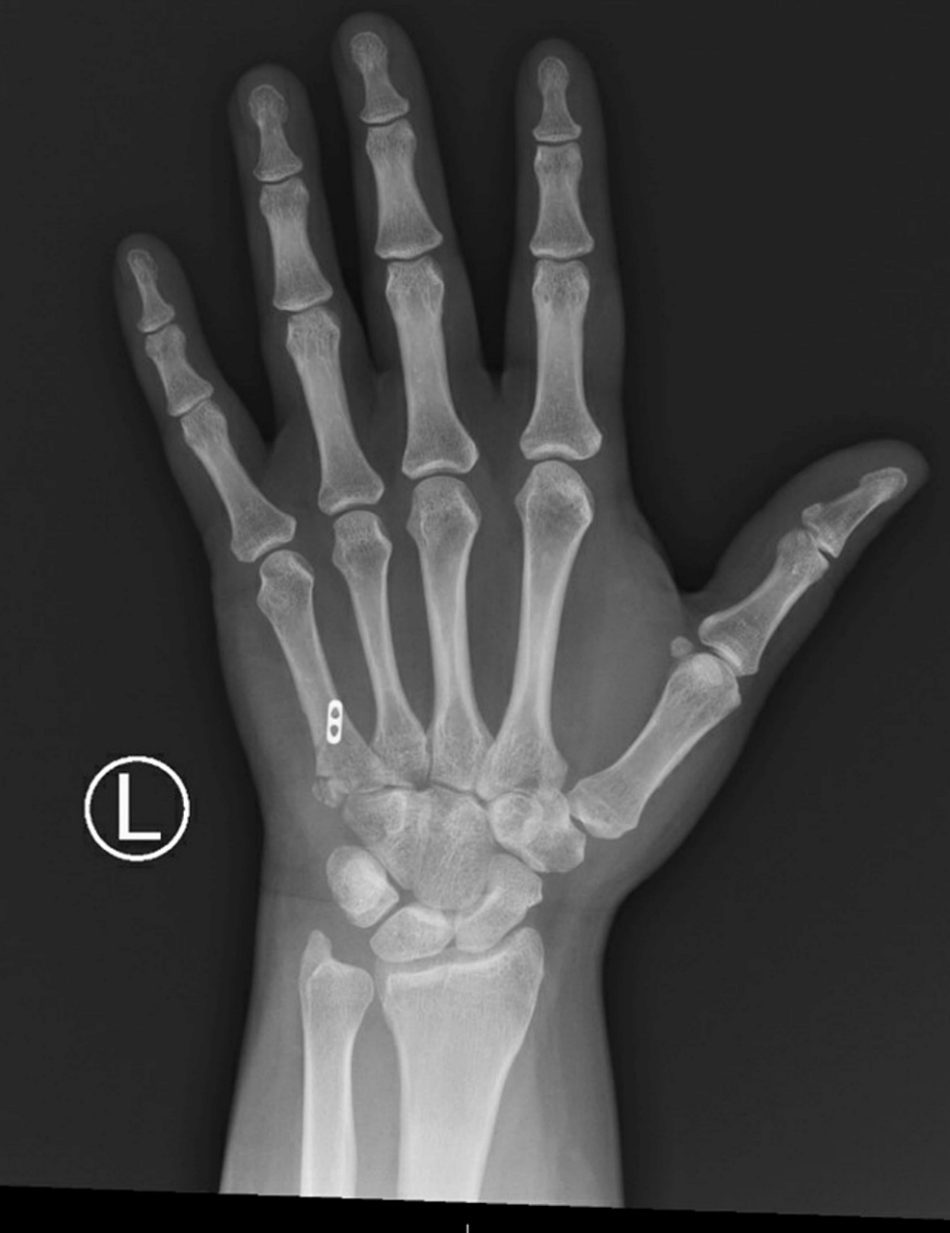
Post-operative (eight weeks) PA radiographs.
